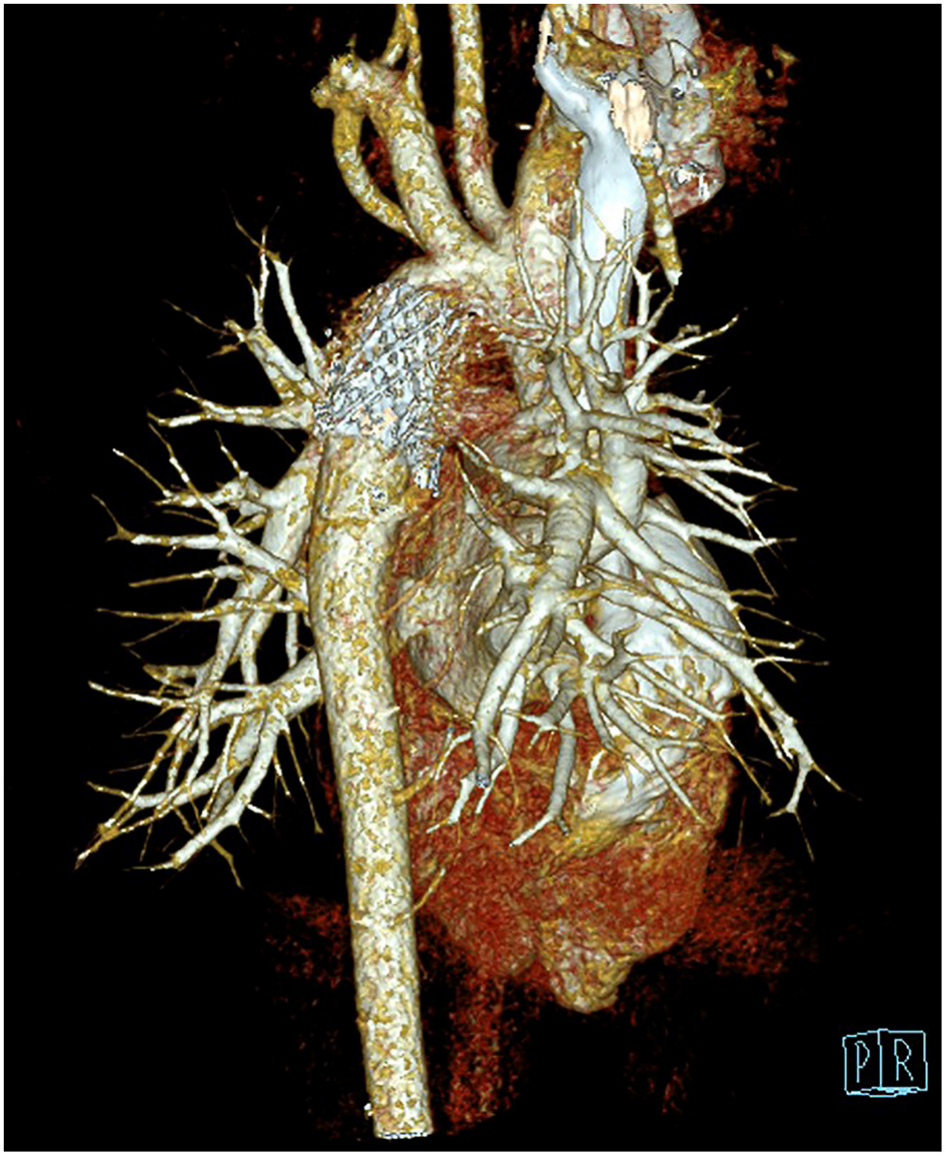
Computed tomography angiography postoperatively showing the coarctated descending aorta after endovascular stent dilatation.
